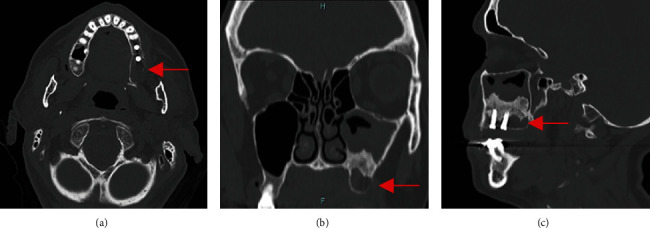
Case 2: (a) axial view preoperative CT scan showing hypodense lesion distal to posterior dental implant (red arrow) and (b, c) coronal and sagittal view showing the lesion (red arrow) developed under sinus lift xenograft.
